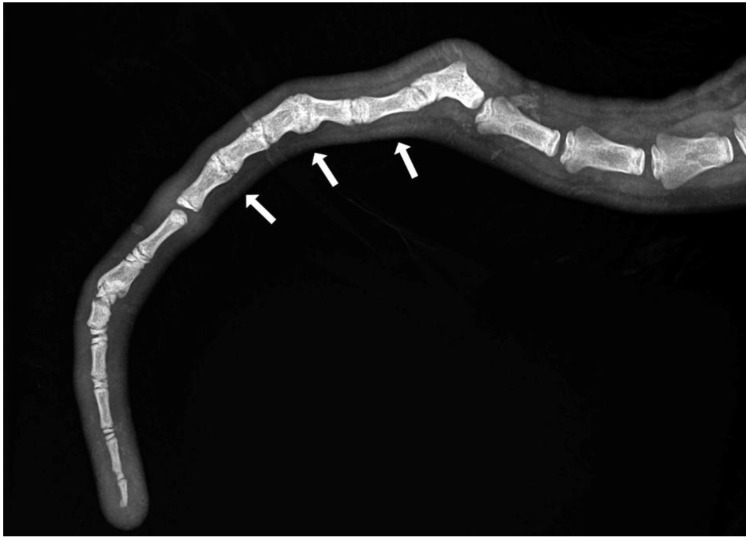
Section of an X-ray of a lamb’s tail with multiple block vertebrae.
